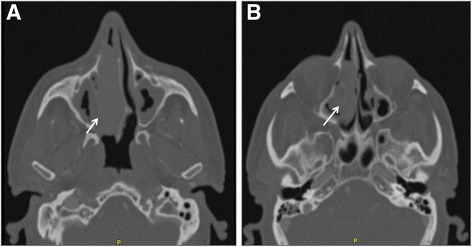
Computed tomography of Case 2. Computed tomography scan, axial view, revealed a soft tissue involving the right nasal cavity (a; *white arrow*), extending into the ipsilateral ethmoid sinus (b; *white arrow*). No signs of bone erosion were observed
